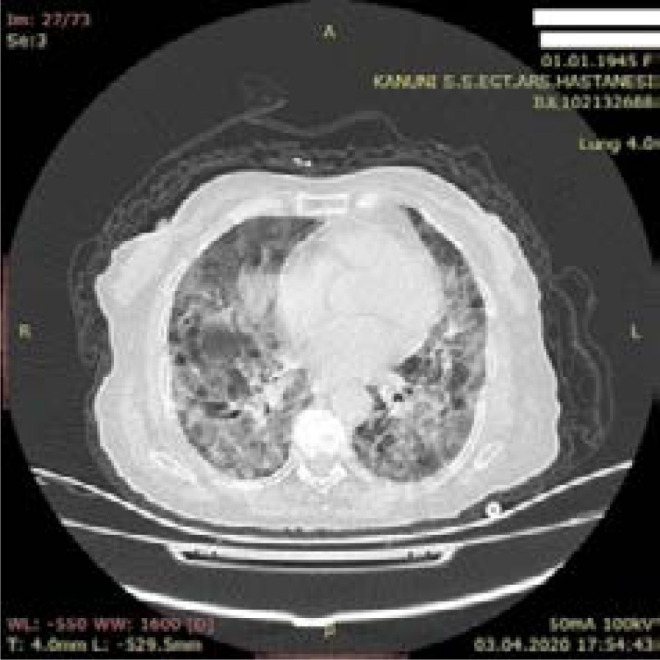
Typical - (Severe) CT finding - 75-year-old female patient - Dead - Multiple patchy ground glass densities combined at places in both lungs
